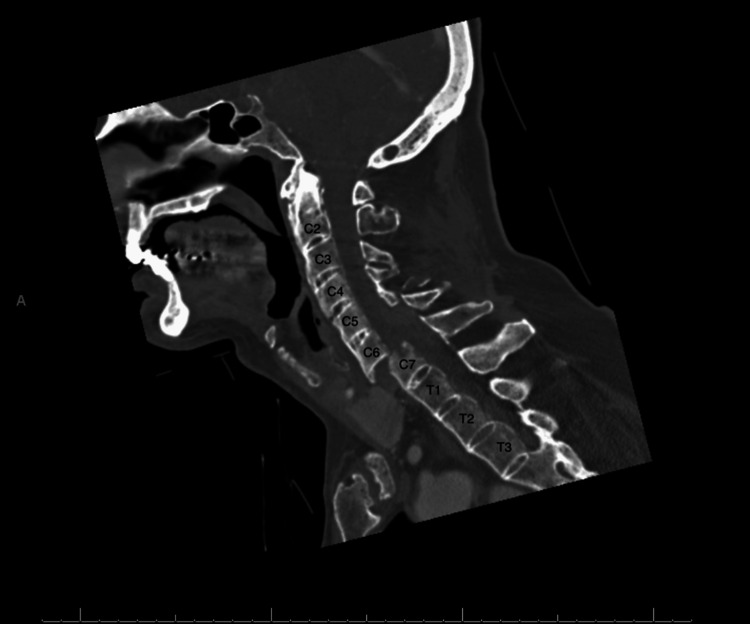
Computed tomography (CT) scan of the cervical spine showing anterolisthesis of C6 on C7 with a posterior epidural hematoma.
